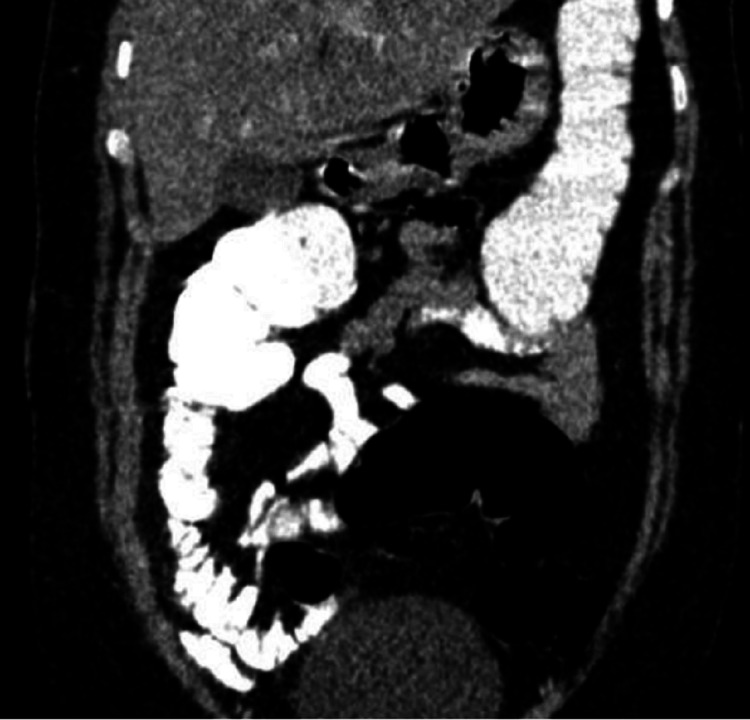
Abdominal/pelvic CT scan with contrast after one month indicating clear appearance without pathology
